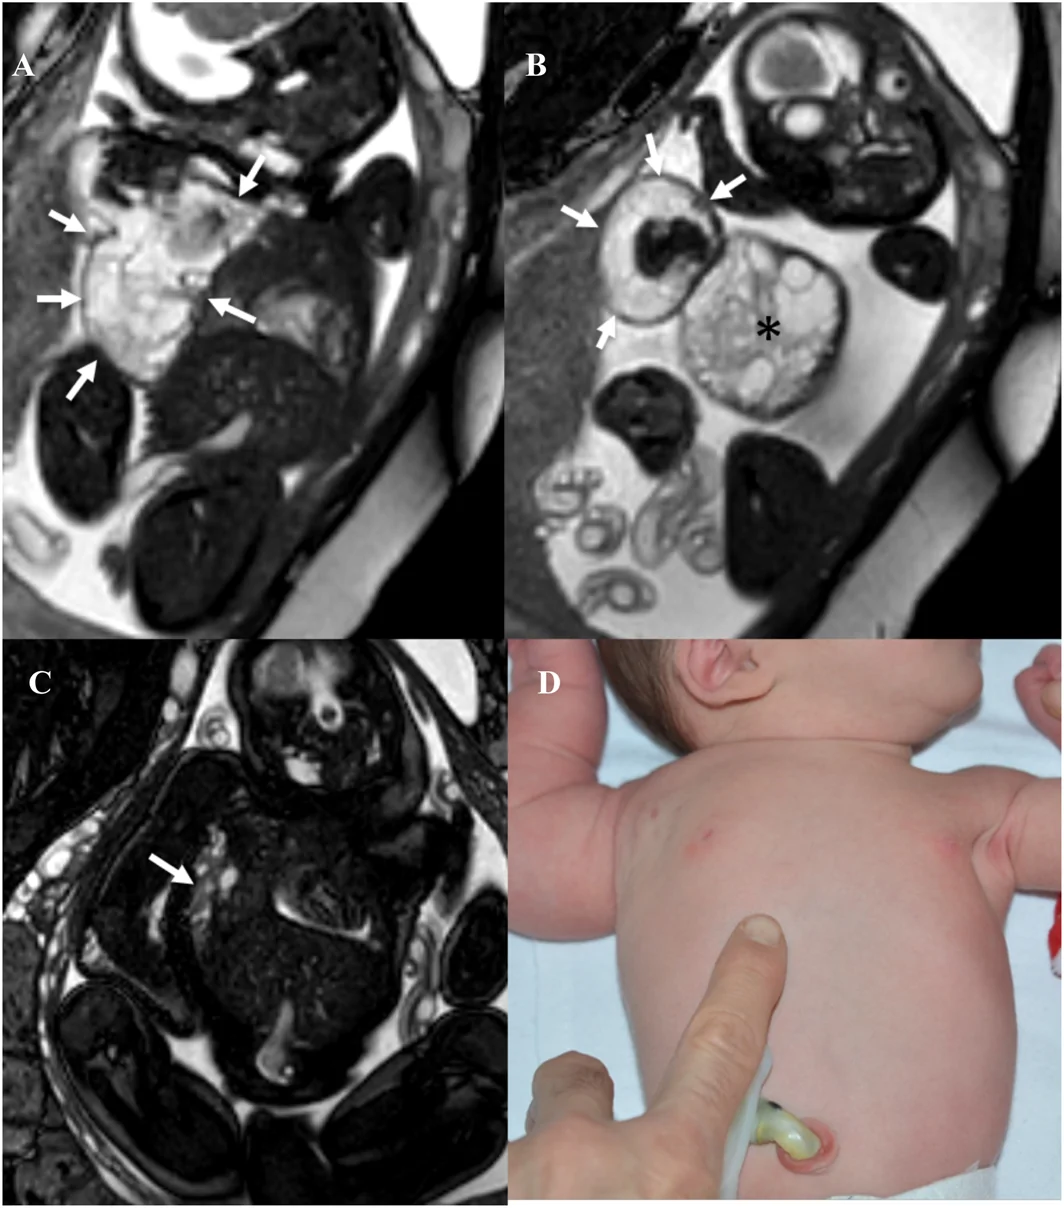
(A–D): Case 4 with MST at 27 gestational weeks with an excellent outcome (graded 4). Coronal T2 weighted coronal MR images (A, B more anterior) showed an extensive LM involving the whole hemithorax with inferior extension facing the right liver (A, white arrows) with a major extension on the anterior thorax (B, black *) and on the right arm (B, white arrows). MR follow‐up at 37 weeks showed a major decrease in the lesion (C, arrow), which was confirmed at birth (D).
